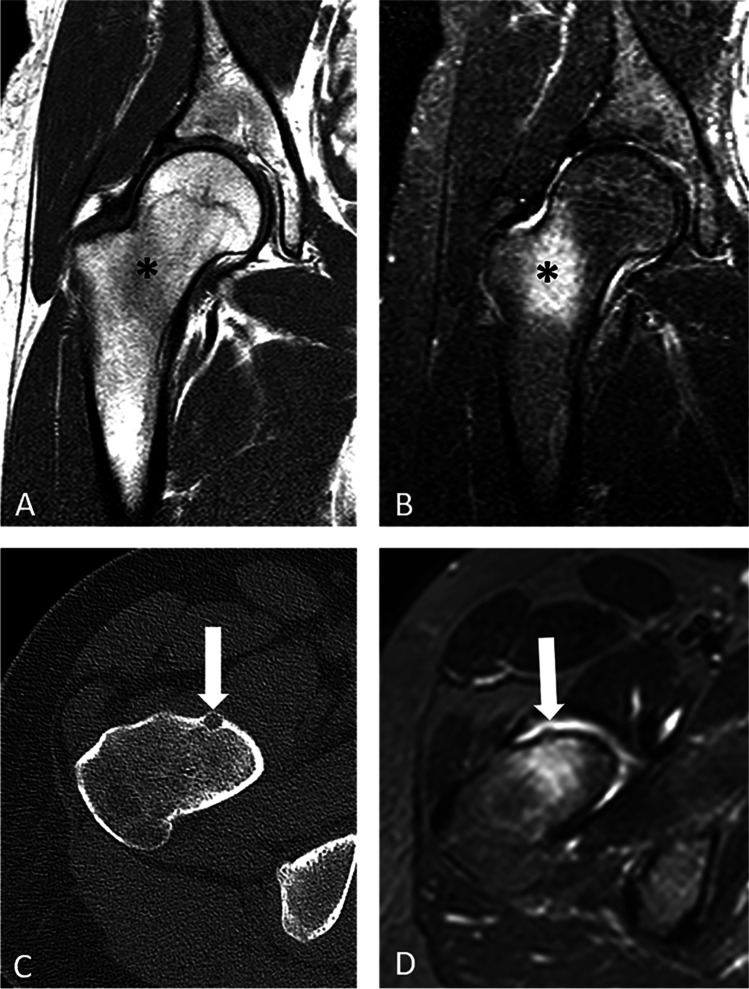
A 19-year-old female with OO of the right femoral neck. Radiographs of the right hip were negative (not shown). Coronal T1 (A) and fat-suppressed T2-weighted (B) MR images show bone marrow edema in the femoral neck (asterisk). She was initially diagnosed with a femoral neck stress fracture, then with FAI, and was sent for metabolic bone disease evaluation prior to being diagnosed with a 6 mm OO that was confirmed on axial CT (C), In retrospect, the OO was evident on the axial T2 weighted MRI (D), but easy to miss if not entertaining the diagnosis (arrow)
